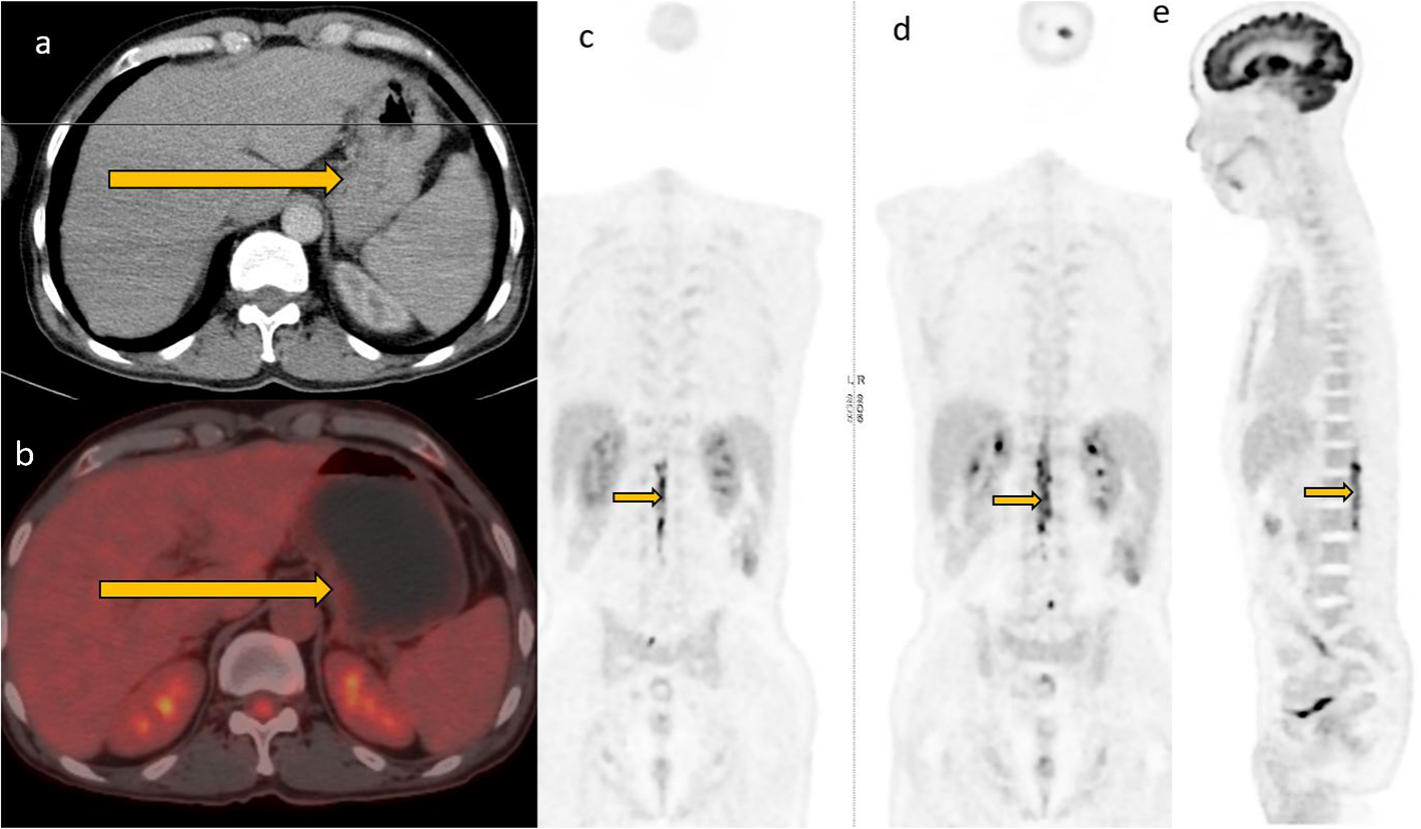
Newly diagnosed HIV-positive patient who had CD4 count of 151 presented with primary gastric lymphoma. He was treated with R-CHOP regimen complicated by severe neutropenic sepsis. Intrathecal chemotherapy was deferred to cycle 3 due to poor performance. Interim PET/CT showed good treatment response of the gastric tumor but there was refractory neuraxial disease seen as FDG avid conus medullaris
